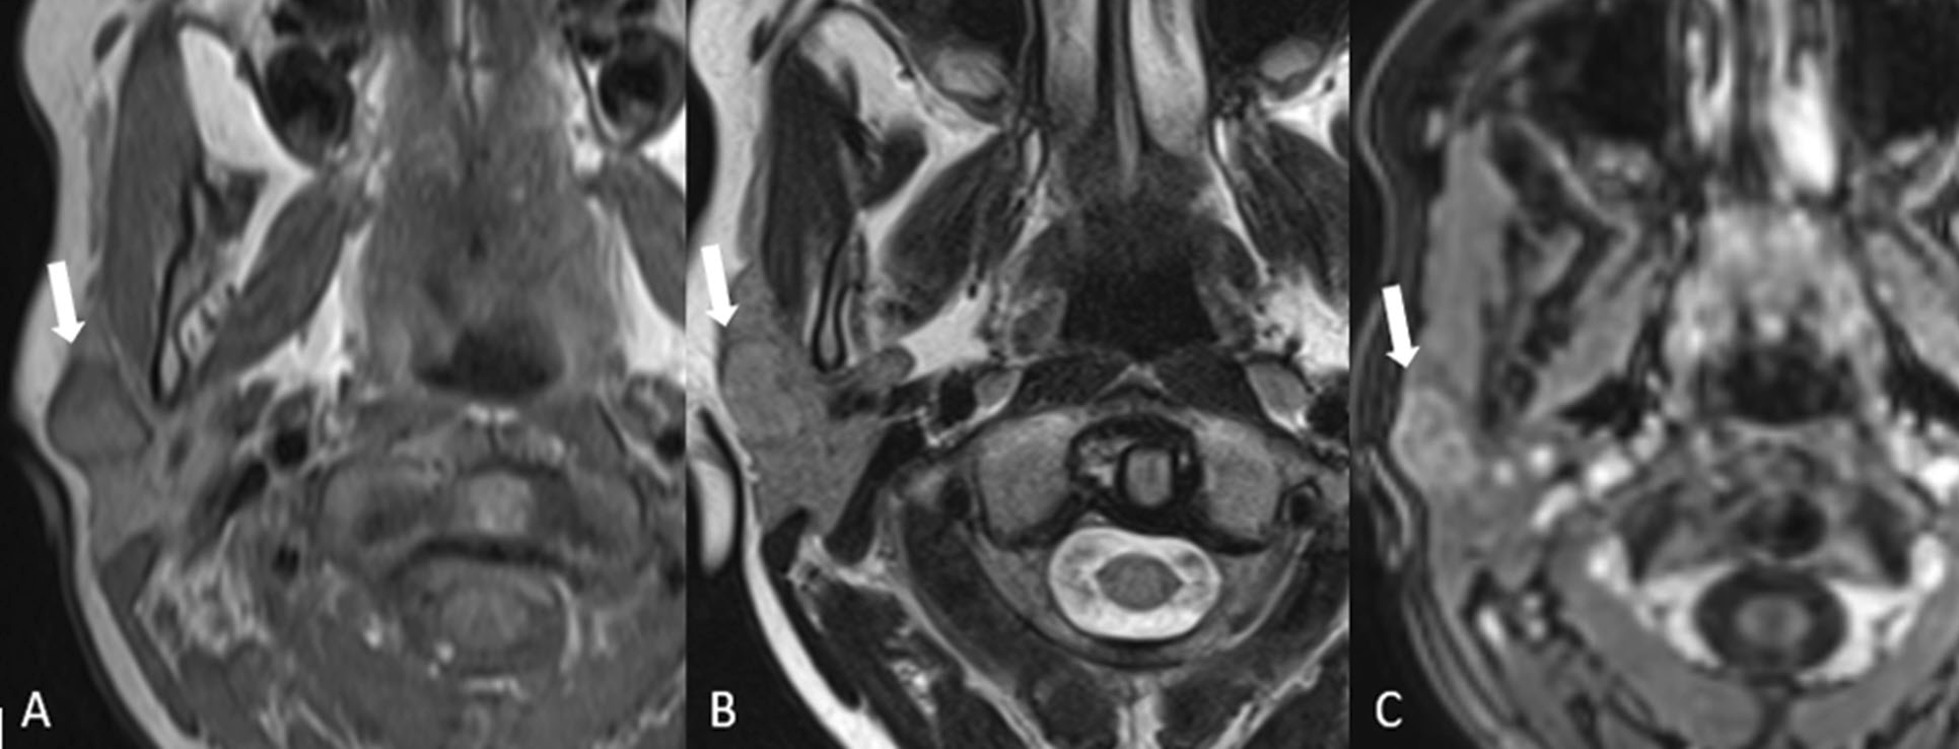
Acinic cell carcinoma. A 10-year-old female with a painless and slowly growing palpable parotid mass on the right side (white arrows) with the clinical suspicion of a lateral neck cyst. Axial T1-w MRI (A) shows a well-defined lesion in the right parotid gland relatively hypointense to the parotid tissue. The lesion is only slightly brighter than the parotid tissue in the axial T2-w (B) MR image. There is some heterogeneous enhancement on the T1-w fat sat MRI after administration of contrast (C)
